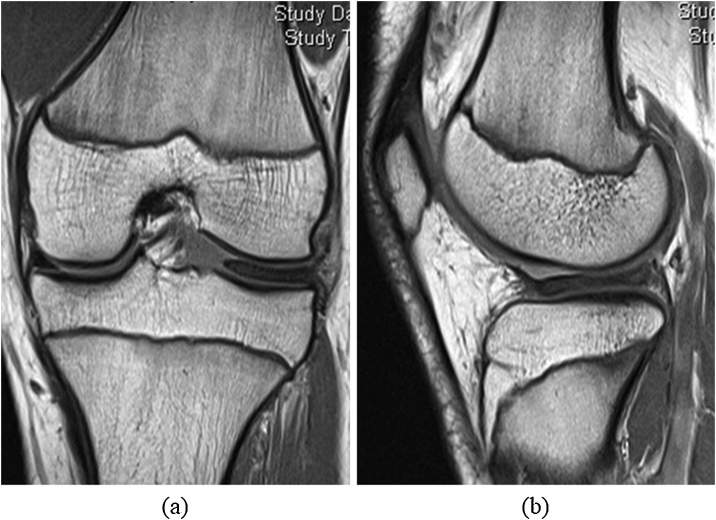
MR images before first arthroscopic surgery for the left knee. Coronal (a) and sagittal (b) proton density-weighted images show a complete discoid lateral meniscus. Growth plates are visible.
